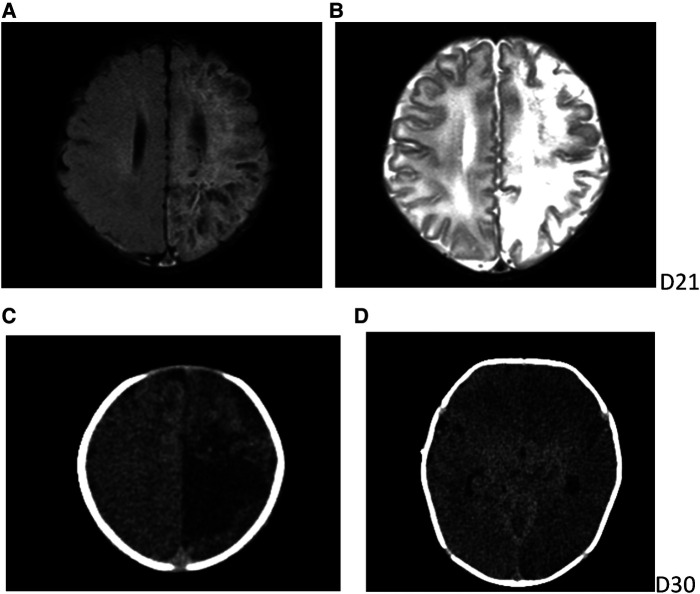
Cranial imaging findings. Routine brain MRI on day 21. (A,B) Diffuse multiple cystic and lamellar abnormal signal areas in the left frontal and parietal lobes with low signal in T1-weighted imaging and high signal in T2-weighted imaging, with speckled diffusion restriction within the lesion. Urgent cranial CT on day 30. (C,D) Diffuse hypodensity in the left cerebral hemisphere and bilateral cerebellum, with a large patchy hypodense area in the left frontoparietal lobe with a CT value of 10–15 HU and multiple speckled hyperintensities within it, a widened left ventricle and additional patchy hyperintensities in the left cerebellar hemisphere.
